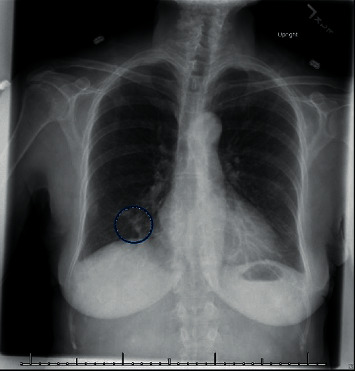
PA chest radiograph showing confluent opacity in the right lower lung.
